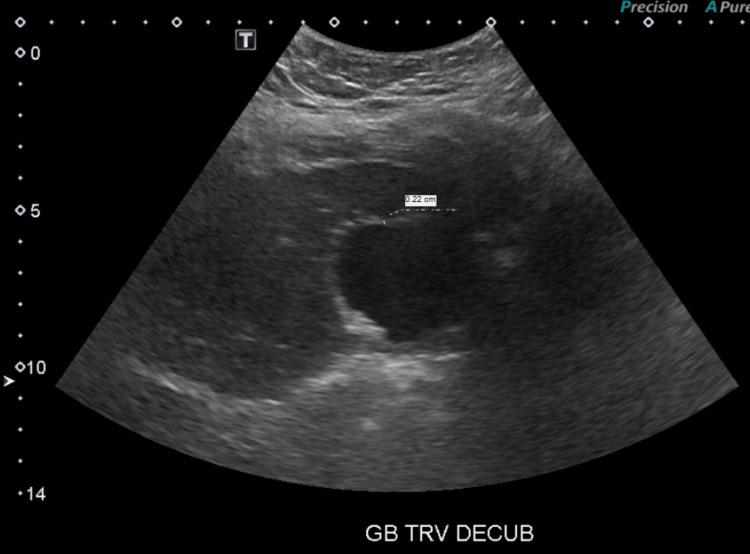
Gallbladder ultrasound in a transverse decubitus position The gallbladder wall measures 2 mm, and there is no pericholecystic fluid.
